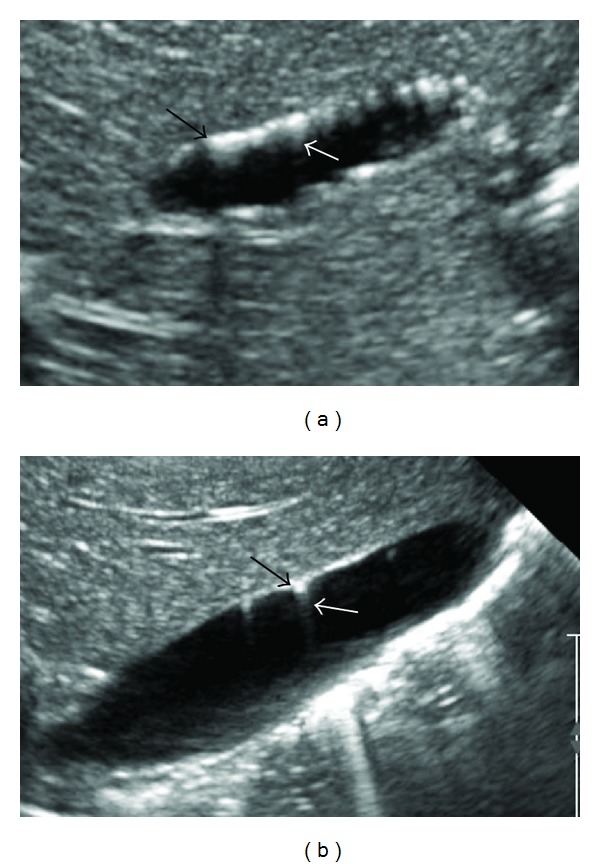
Ultrasound images at 4 months of age (a) and 7 months of age (b). Multiple nondependent echogenic foci were seen in wall of gall bladder or Rokitansky-Aschoff sinuses (black arrow) with distal sonographic shadowing or comet tail artifacts (gray arrow).
